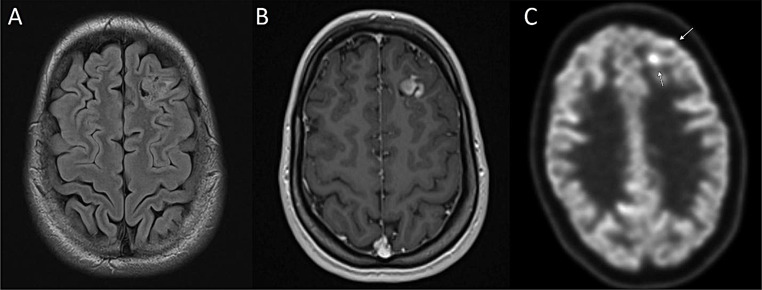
Patient imaging. A 24-year-old woman who initially presented with a bilateral tonic-clonic seizure and found to have a left frontal enhancing lesion. Panel A shows an axial T2 FLAIR image showing a 1.8 x 1.5 x 2.3 cm lesion within the cortex bridging the left superior frontal sulcus. Panel B showed axial T1 post-contrast imaging showing a 2.3 cm heterogeneously enhancing lesion centered along the cortex bridging the midportion of the left superior frontal sulcus. Panel C shows PET CT scan with focal hypermetabolic activity in the left anterior frontal lobe corresponds to the enhancing components of the 2.3 cm lesion in this location on prior brain MRI (arrow). Focal decreased activity just superior to the above-described region in the left frontal lobe cortex (arrow)
